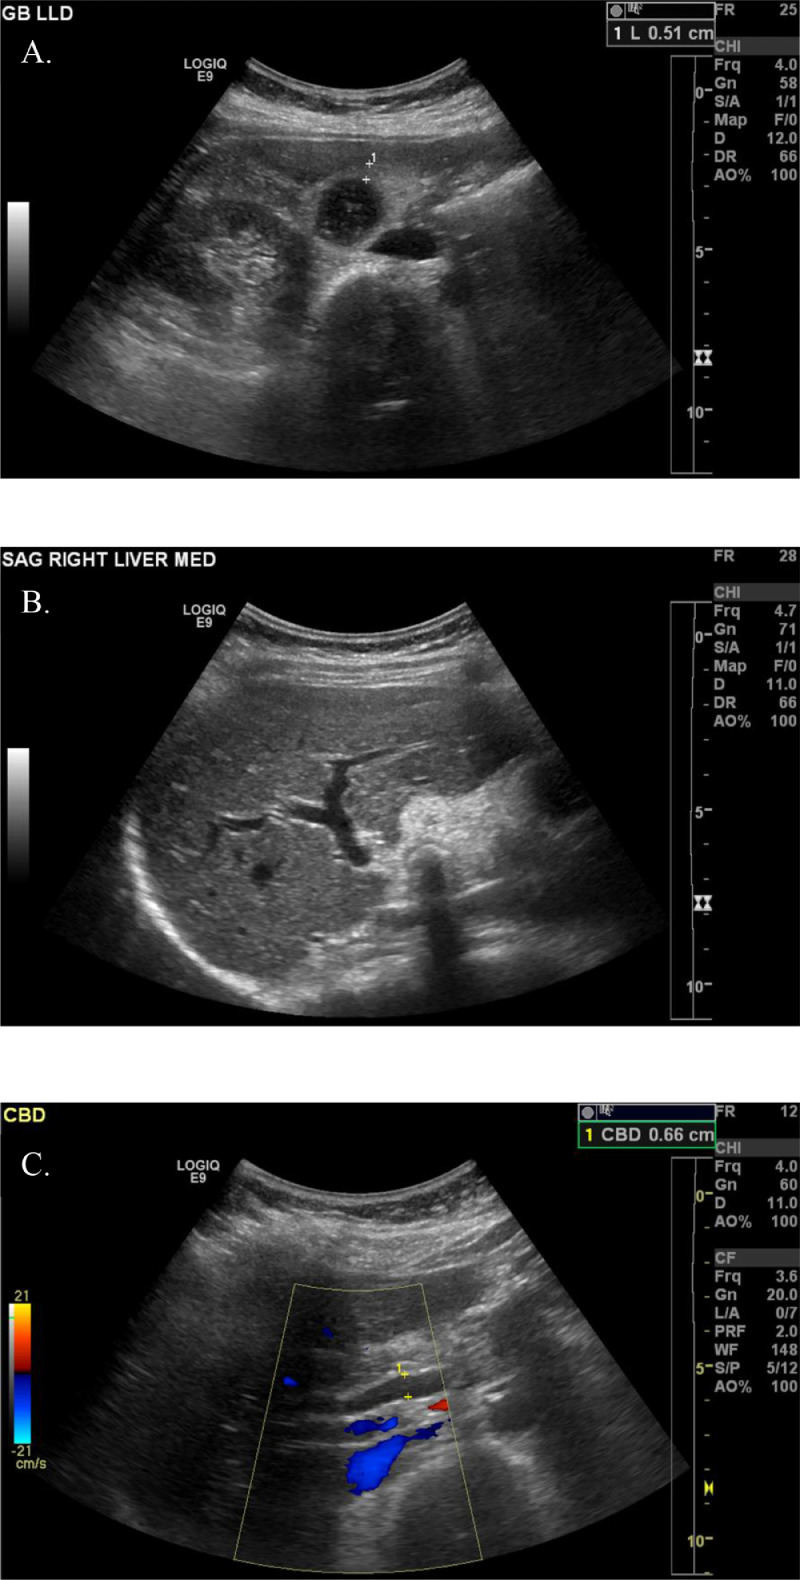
Abdominal ultrasound. Abdominal ultrasound revealing (A) thickened gallbladder with cholelithiasis, (B) intrahepatic biliary ductal dilation, and (C) extrahepatic biliary ductal dilation.
